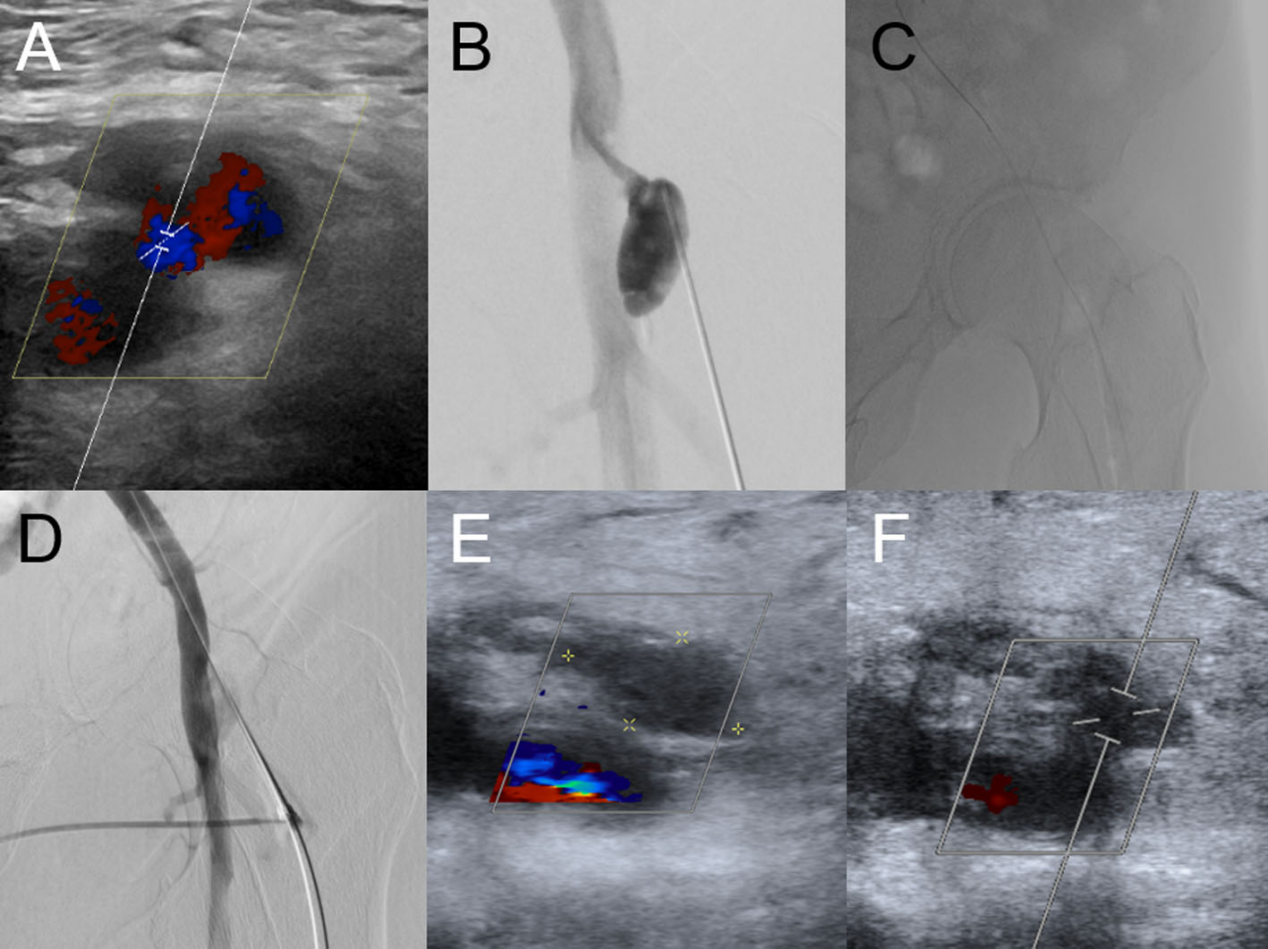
Well-perfused PSA of the CFA in a 91-year-old patient (Patient No. 3) after TAVI. A Sonography showing persistent perfusion after single thrombin injection. B Puncture of the PSA close to its neck and DSA via the connecting catheter to visualize the patient’s vascular anatomy. C Correct probing and positioning of the microwire in the pelvic axis. D Insertion of a 5 F sheath after changing to a 0035’ wire and DSA showing only residual perfusion of the PSA, confirming correct position. E and F Sonography on the next day shows nonperfused PSA the next day
